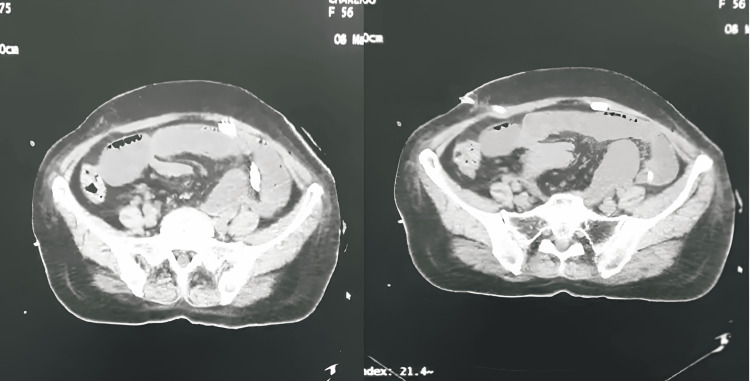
Axial slice of an abdominopelvic CT scan with intravenous contrast at the abdominal level, showing a mechanical small bowel obstruction associated with an abdominal drain.
